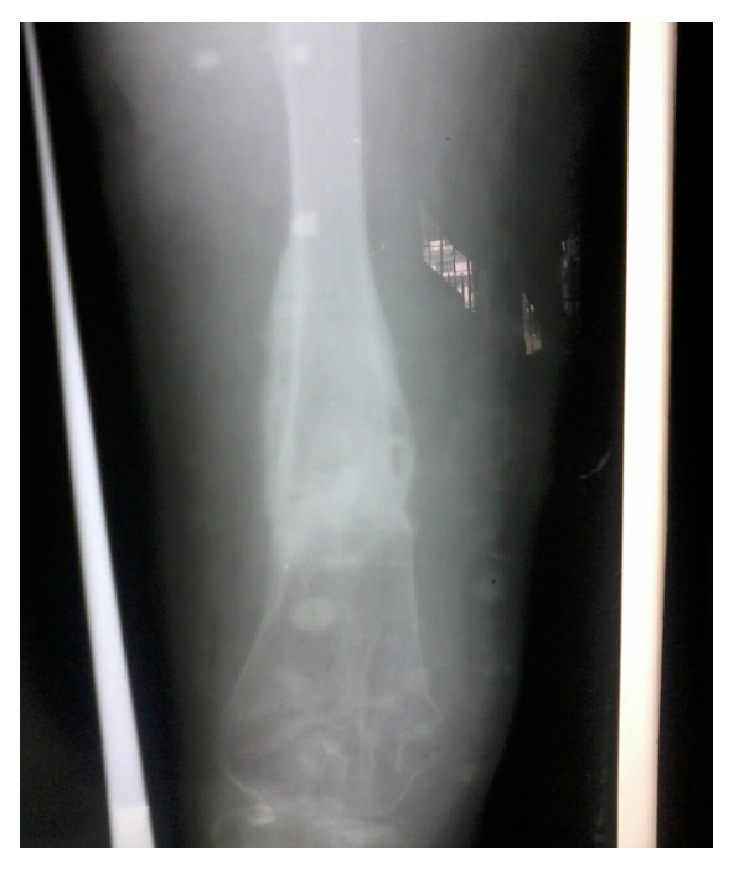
X-ray four and half months postoperative anteroposterior view, showing fracture consolidated.
